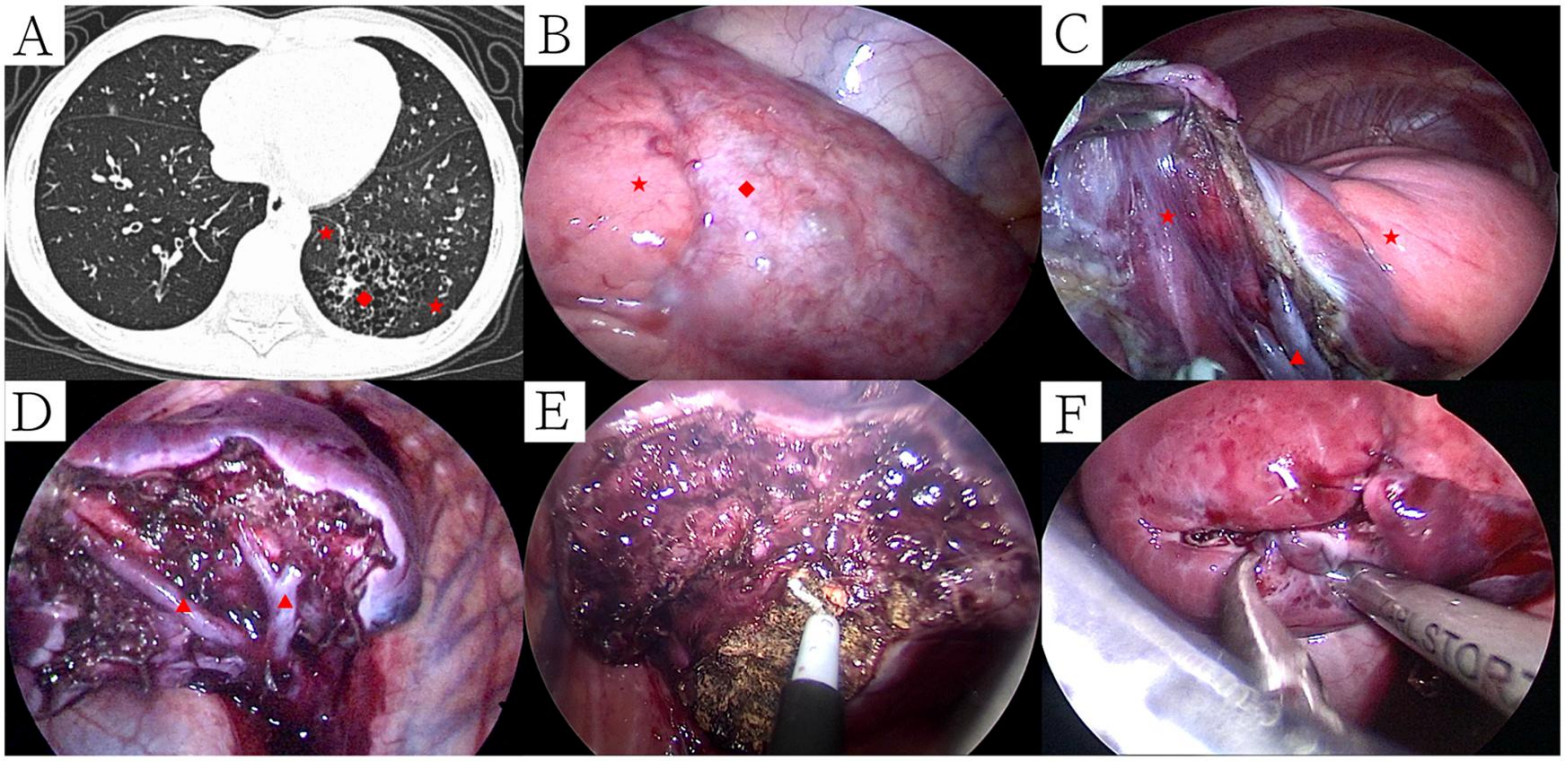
Ct manifestations and intraoperative management of CPAM patients with recurrent pulmonary infections recently. (A) CT manifestations of CPAM patients with recurrent pulmonary infections recently (lung window); (B) External boundary between healthy and affected lung tissue; (C) Cut and separate along the boundary between CPAM and normal lung tissue; (D) Internal boundary after removal of CPAM (segmental veins); (E) Identified bleeding sites were meticulously cauterized with the electric hook; (F) Resection margins were reinforced using continuous 5-0 PDSII sutures. Symbols: CPAM: ◆, Normal Lungs: ★, Intersegmental vein: ▴.
